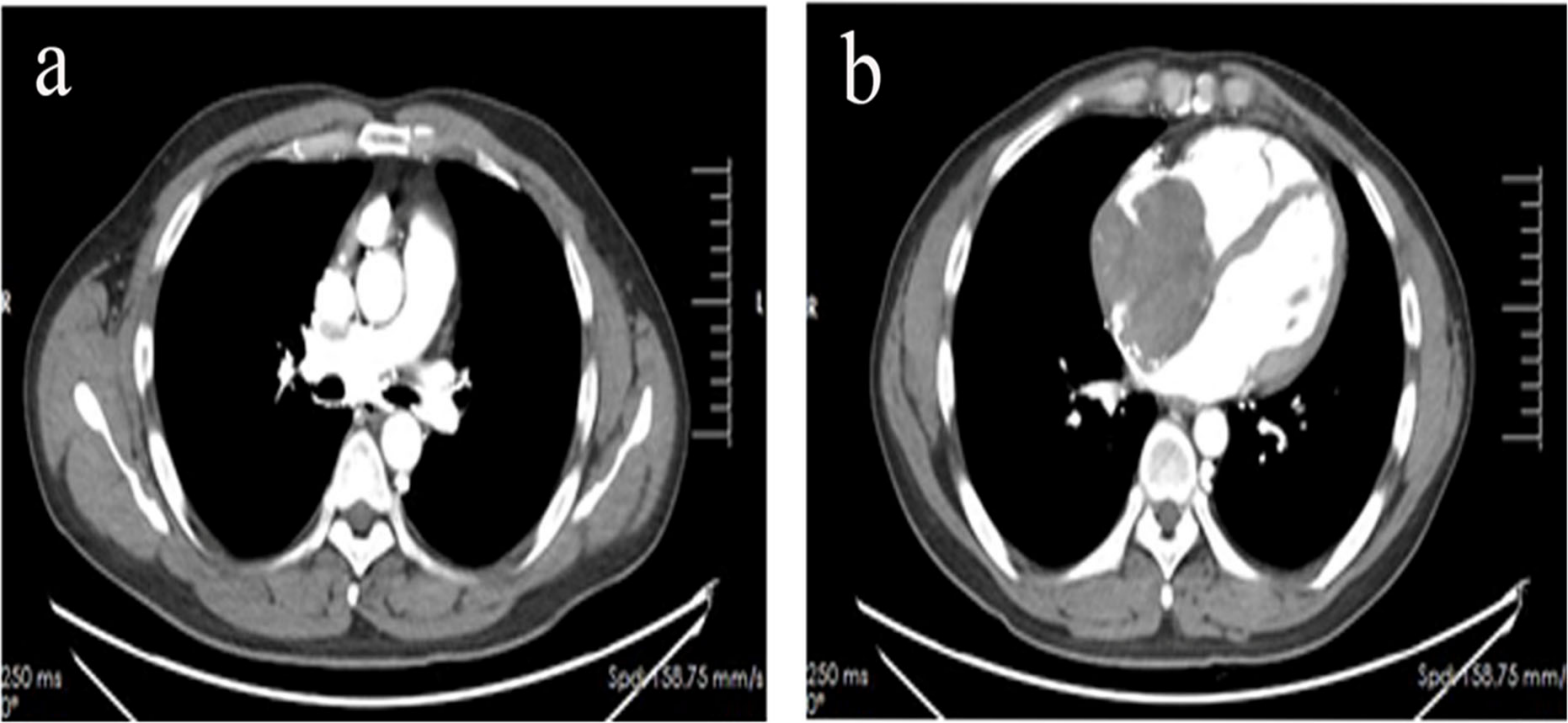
CT venous angiography revealed filling defects in the superior vena cava (a) and right atrium (b).
